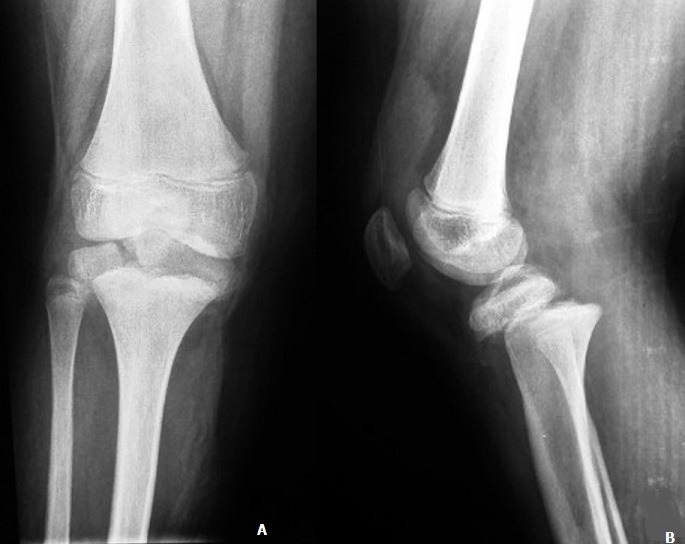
(A) anteroposterior radiograph of the right knee demonstrating Salter-Harris III fracture fragment; (B) lateral radiograph of the right knee demonstrating Salter-Harris I fracture fragment and anterior displacement of the epiphysis
